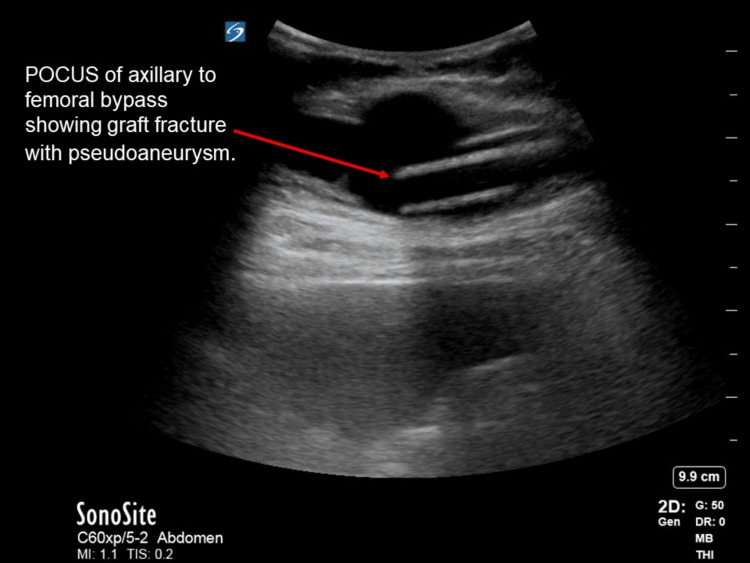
Sagittal and sonographic image of the vessel with the graft fracture.
